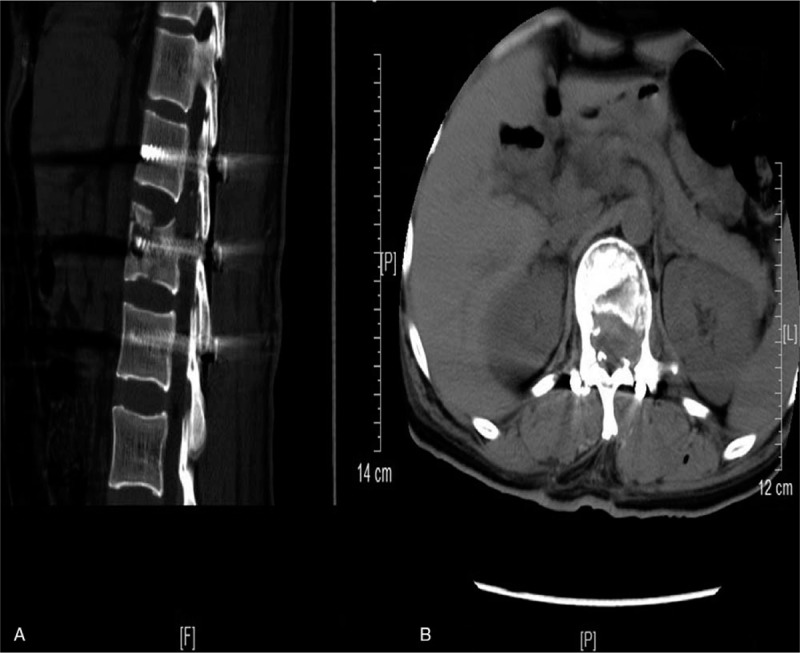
The images of the patients were re-examined on the 3rd day after the second operation. The sagittal (A) and axial (B) of computed tomography showed no residual bone fragments in the spinal canal and complete decompression of the spinal cord.
